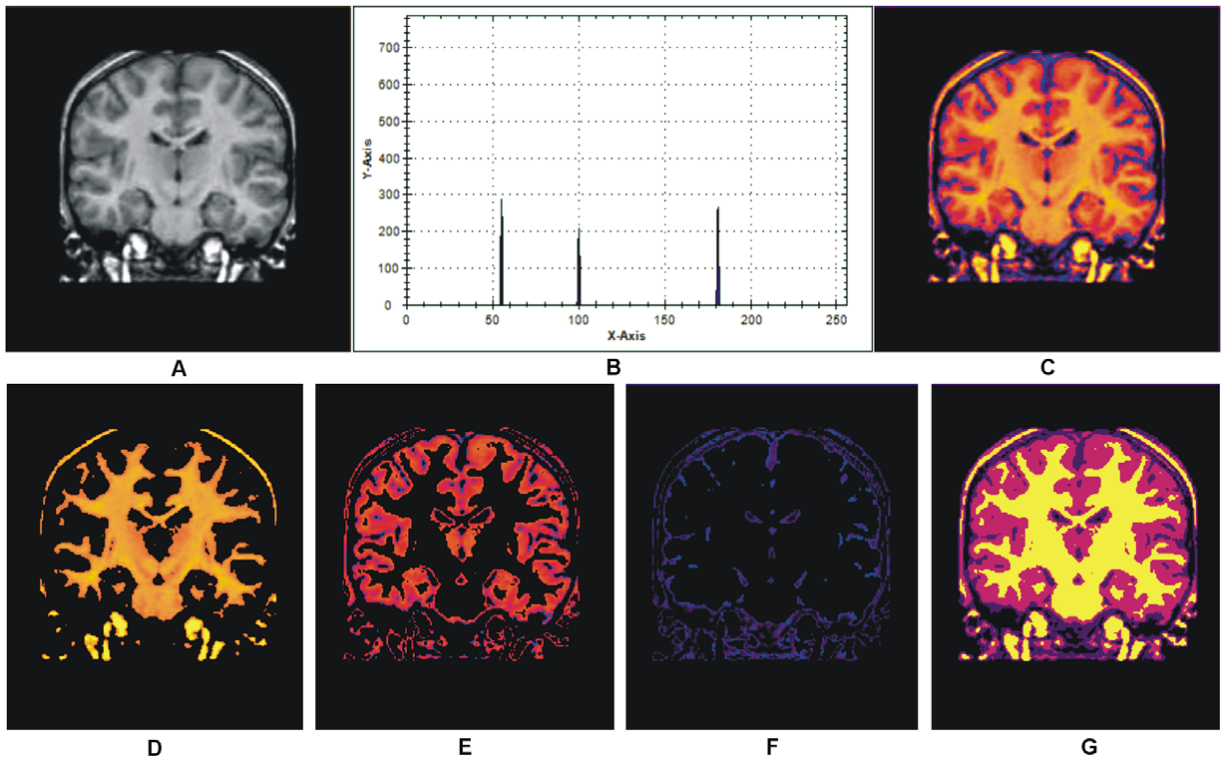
Results of our proposed colorization and segmentation method on T1 brain MR image. (A)T1 Brain MRI image [54]. (B) Selected centroids with the proposed auto centroid selection method. (C) Color transformed image of (A) with proposed colorization method. (D)–(E) Extracted matter one, extracted matter second and CSF respectively with proposed segmentation method utilizing the selected centroids shown in (B). (G) Whole segmented image with proposed method.
